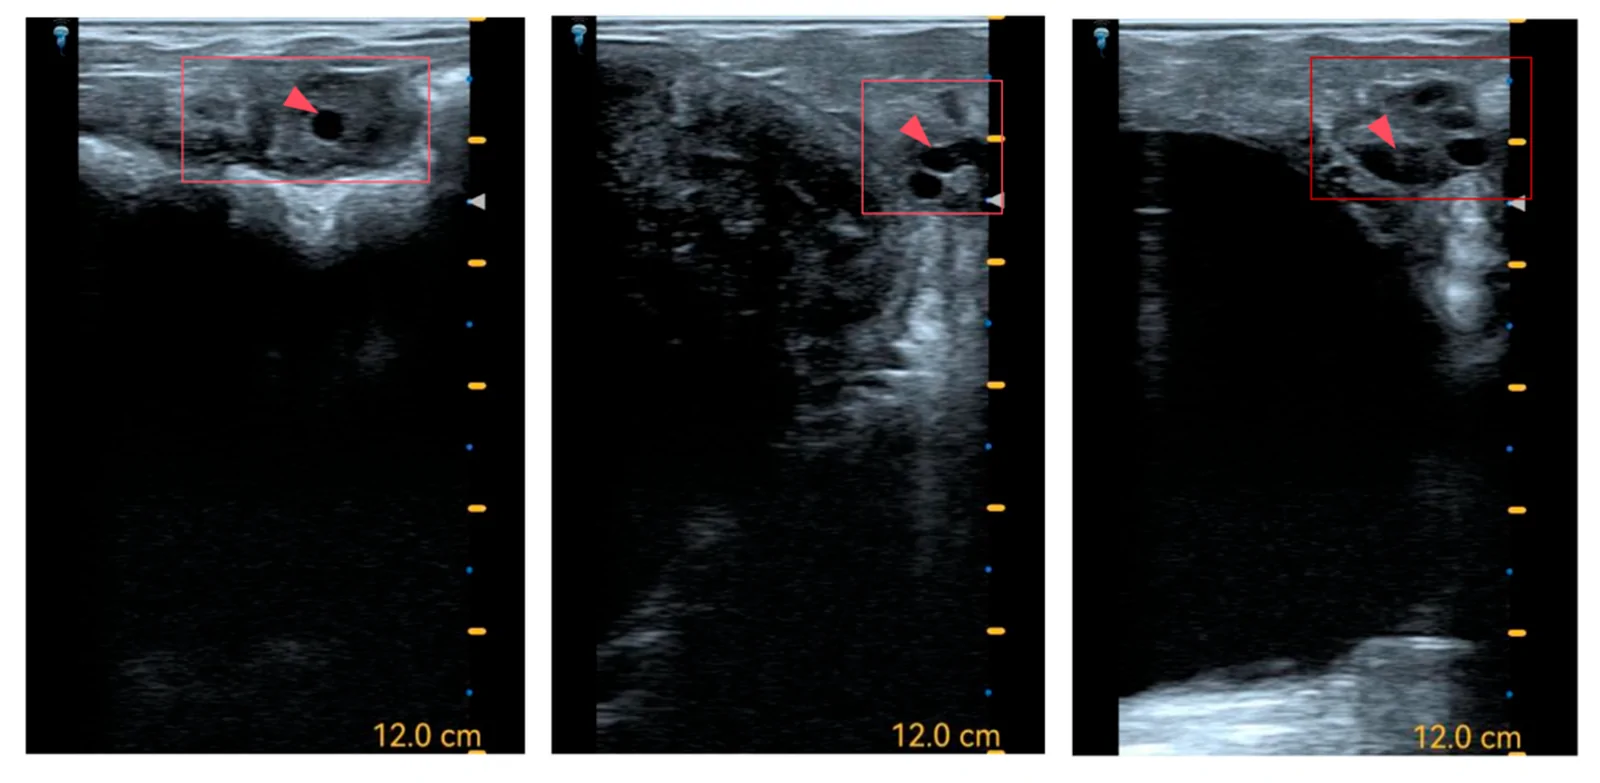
Images of growing follicles in camels detected by Ultrasound B. Growing follicles are shown in the red boxes.
